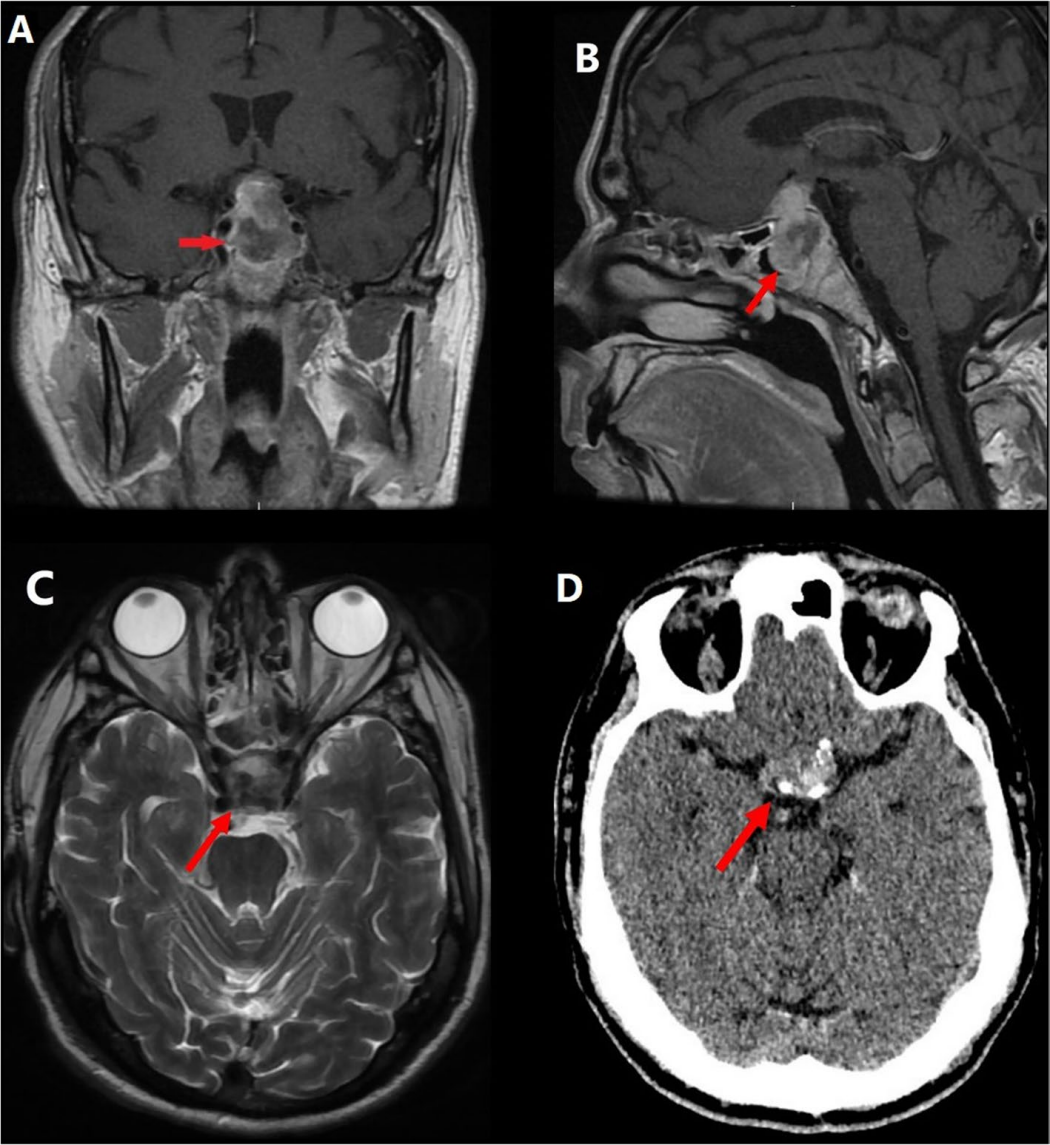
Subsequent imaging 3 months later demonstrating pituitary apoplexy. A T1 coronal MRI showing pituitary macroadenoma (2.6 × 2 × 2.3 cm) with evidence of apoplexy and stretching and compression of the optic chiasm. B T1 sagittal MRI with contrast. C T2 axial MRI without contrast. D non-contrast CT head
